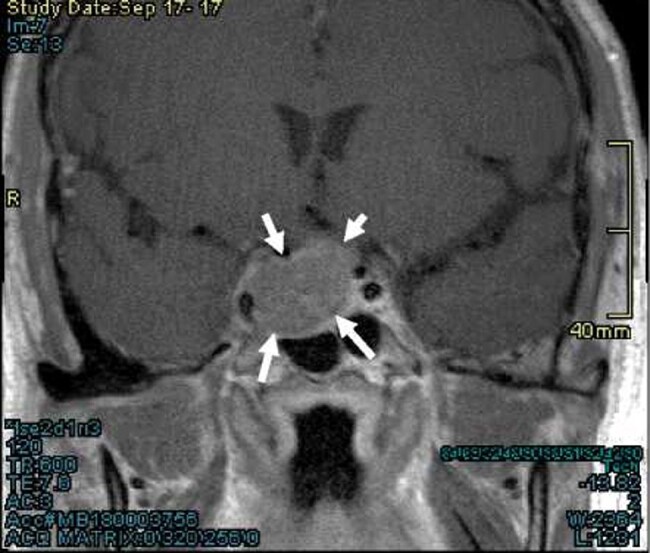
Coronal T1 view of magnetic resonance imaging sella at initial presentation showing a 2.0 × 2.2 × 3.1 cm central lesion with extension to the right cavernous sinus and suprasellar cistern reaching the optic chiasm.
